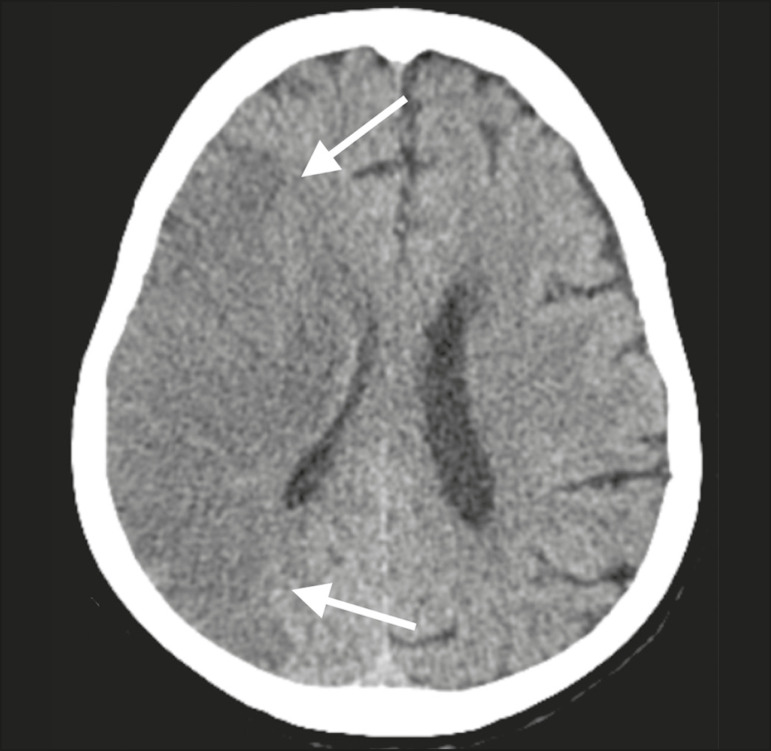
Case 1. Brain CT of an 80-year-old woman with COVID-19 showing an extensive acute ischemic stroke, affecting the right MCA territory (arrows).
